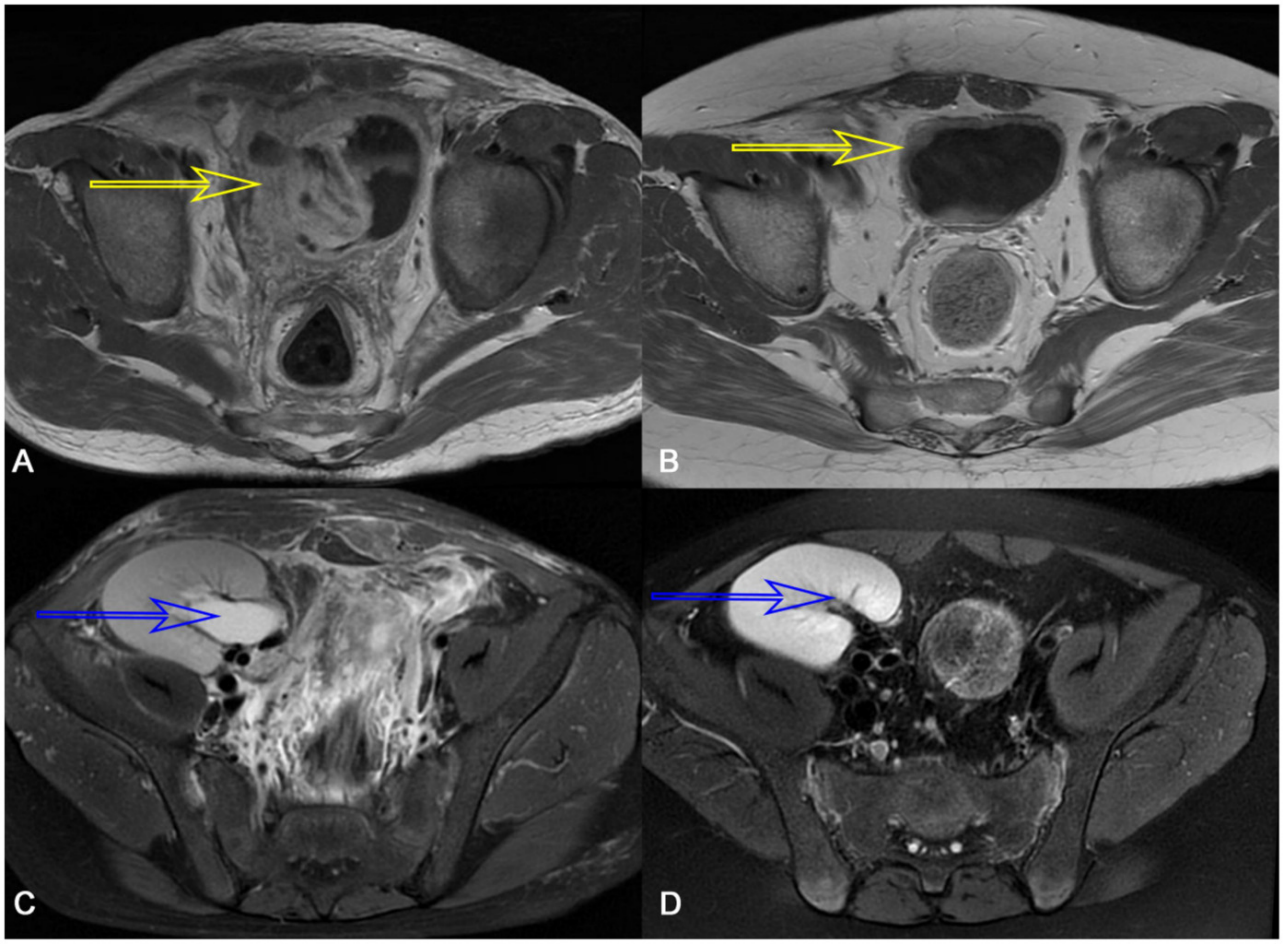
CT scan showed thickened bladder wall (yellow arrow) before treatment (A) and after treatment (B) and graft kidney hydronephrosis (blue arrow) before treatment (C) and after treatment (D).
